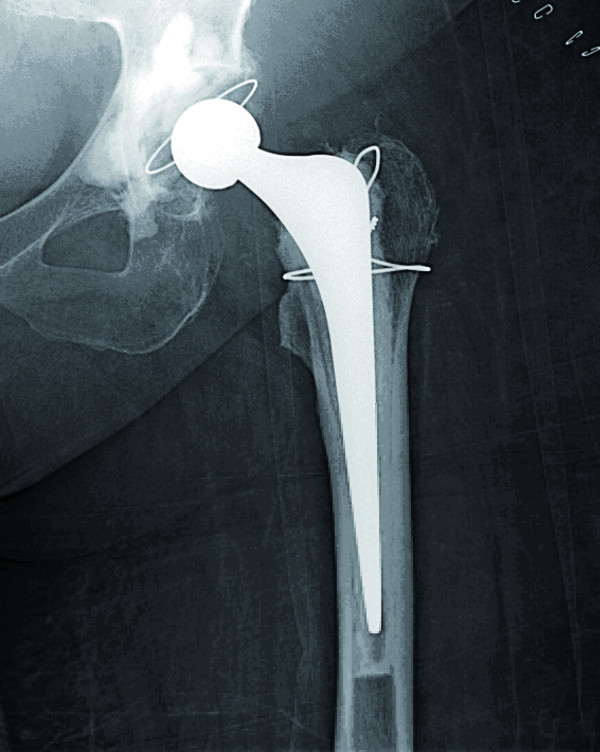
Anteroposterior radiograph showing the cemented total hip prosthesis with no obvious cement extrusion in the pelvis or soft tissue.
